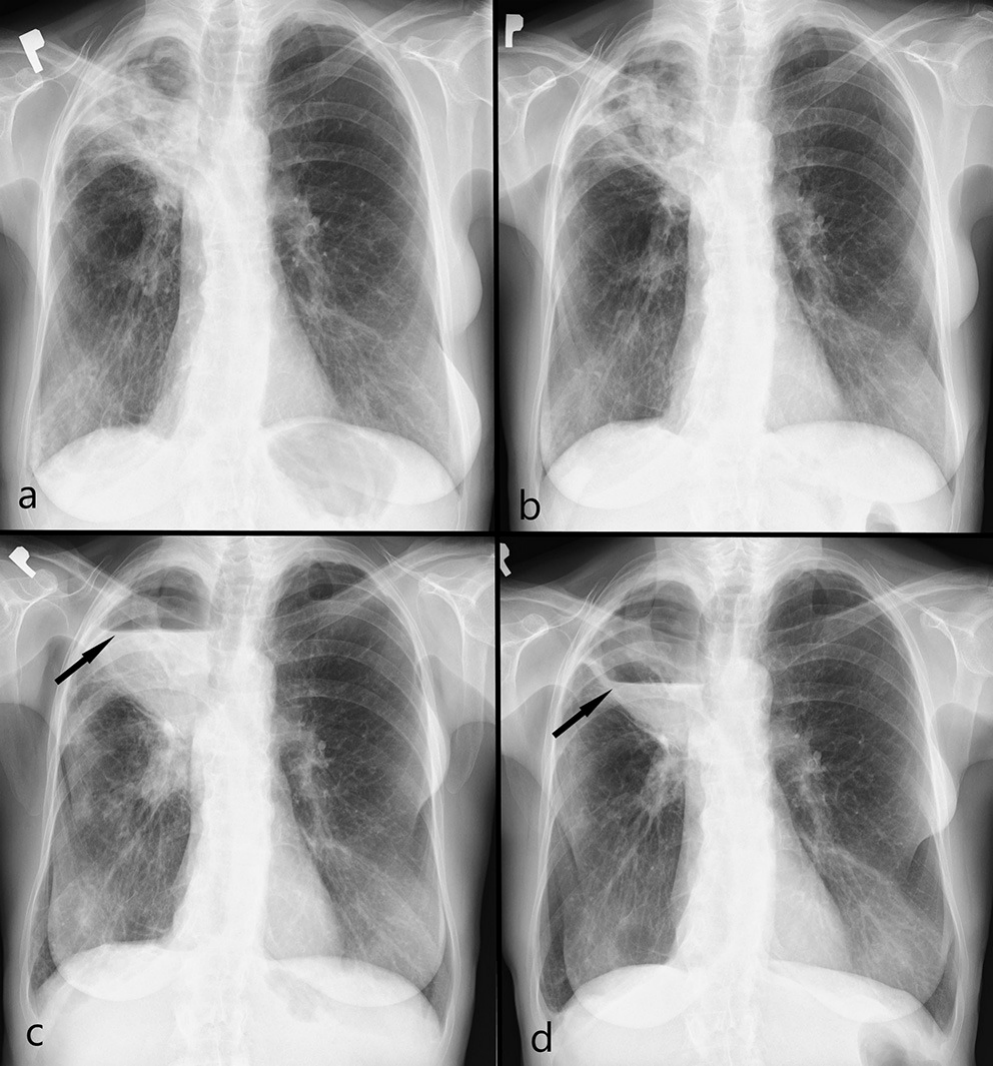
Controlled chest x-ray during second-line treatment in 2021: (a) at the beginning of the therapy - a thick-walled cavity in apical parts of the right lung, progression internal consolidations and surrounding opacities; (b) after 3 months – cavity consolidation, regression of surrounding opacities; (c) after 4 months – cystic lesion with an air-fluid level (arrow) (progression, abscess); (d) after 5 months – regression of an air-fluid level (arrow).
